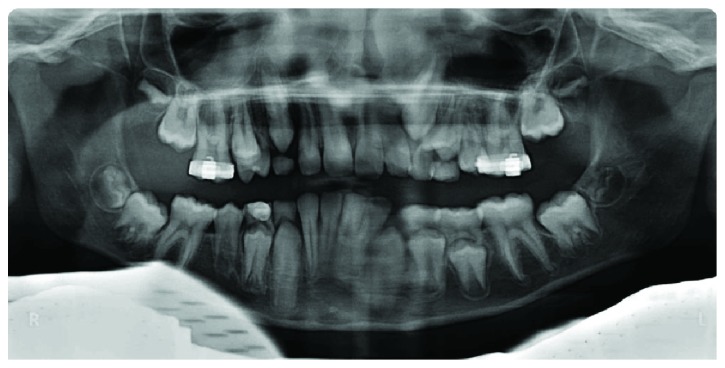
Orthopantomogram of the case at the end of the treatment. The second premolar (4.5) has successfully erupted, and the first molar (4.6) presents a correct inclination.
